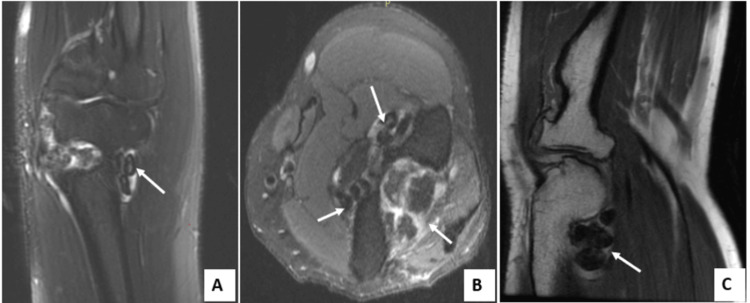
T2-weighted MRI (A) coronal, (B) axial; T1-weighted MRI (C) sagittal Shows joint effusion and multiple hypointense nodules on T1- and T2-weighted sequences, consistent with cartilaginous loose bodies, as well as associated degenerative changes and synovial thickening.
